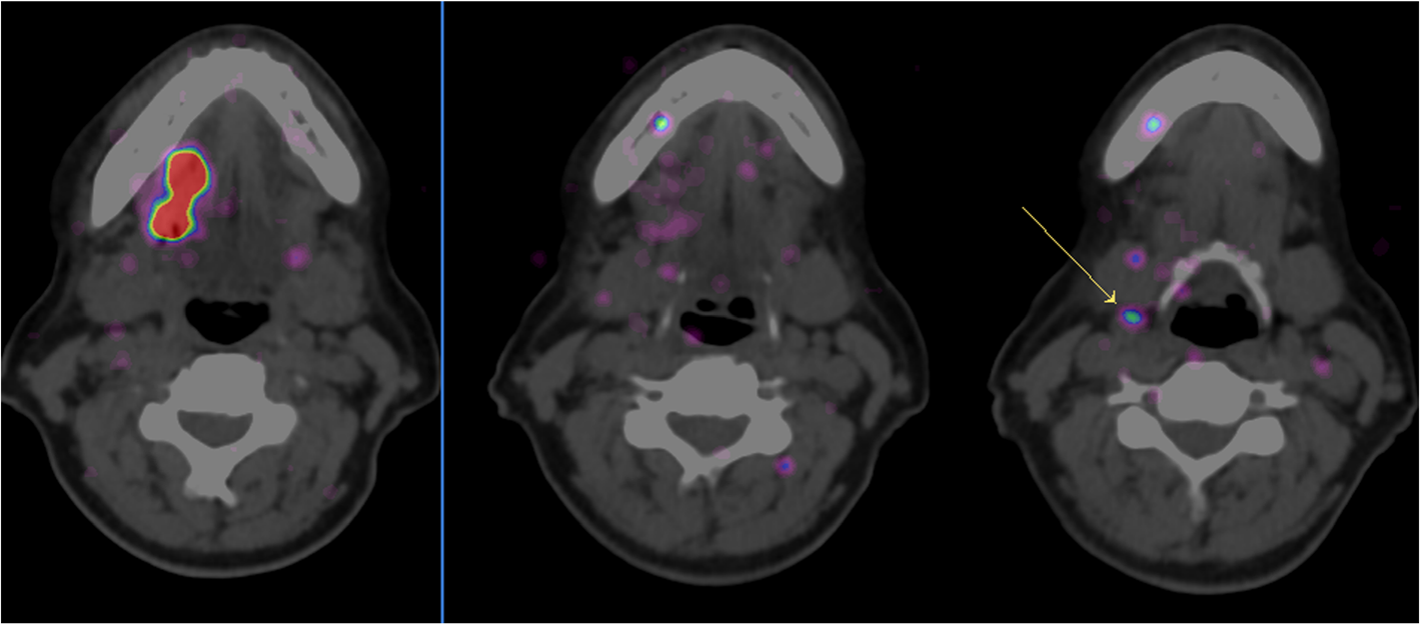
Axial fused PET/CT images of patient 2 showing the injection site of 89Zr-nanocolloidal albumin in the lateral tongue on the right side and low uptake in SLN in level II (arrow)
